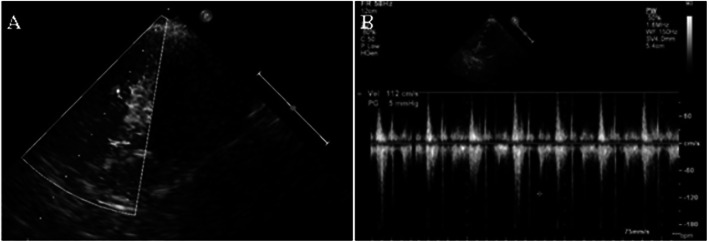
Postoperative Ultrasound (a. No regurgitation or paravalvular leakage was found; b. trans-prosthetic valvular flow velocity was normal)
